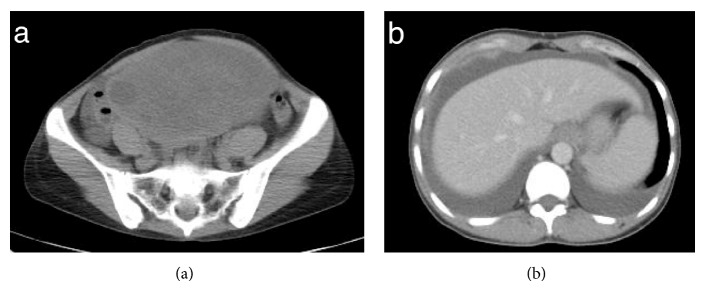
Preoperative computed tomography (CT) findings. (a) CT of the pelvis shows a left ovarian tumor 16 cm in diameter. (b) Bilateral pleural effusion and ascites.
